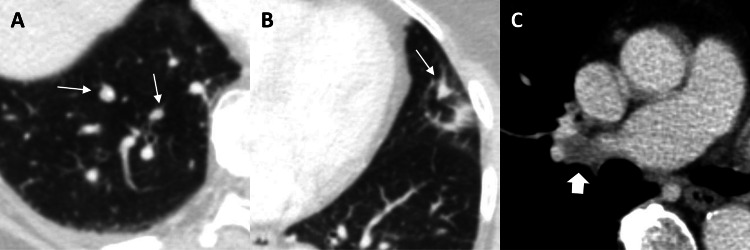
Staging CT. Lung CT shows multiple pulmonary lesions, exemplary in the right (A) and left (B) lower lobes (arrows). There was also a malignant suspicious lymph node in the right hilar region (C, block arrow), which together indicated metastasis. CT: computed tomography
